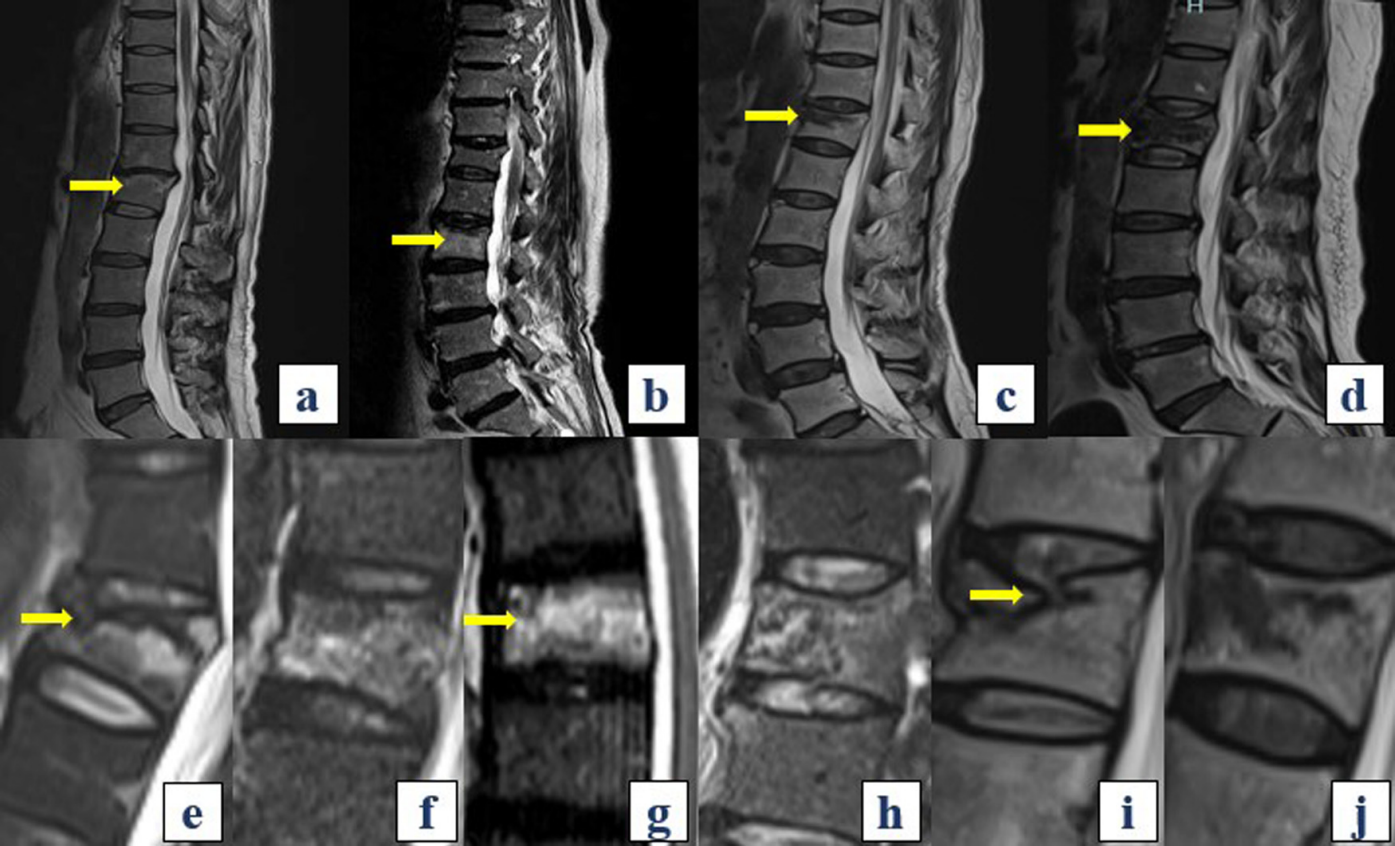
Fracture assessment on magnetic resonance imaging. (a)–(d) Subtyping based on MRI signal changes on T2WI. (a) Type A – confined high signal change (yellow arrow), (b) Type B – diffuse high signal change (yellow arrow), (c) Type C – confined low signal change (yellow arrow), and (d) Type D – diffuse low signal change (yellow arrow). (e) and (f) Typical black line signal change on STIR images. Black line signal is defined as a black line exceeding half of the anteroposterior diameter of the vertebral body on STIR images. (e) Typical black line signal (yellow arrow) and (f) non-black line signal. (g) and (h) Typical homogeneous high signal change on STIR images. Homogeneous high signal is defined as an area of homogeneous high signal exceeding half of the vertebral body area on STIR images. (g) Typical homogeneous high signal (yellow arrow) and (h) non-homogeneous high signal. (i) and (j) Assessment of IDEC integrity on T2WI. (i) Injury to the IDEC above the fractured vertebra (yellow arrow). (j) Intact IDEC both above and below the injured vertebra. T2WI: T2-weighted images; STIR: short tau inversion recovery; IDEC: intervertebral disc endplate complex.
